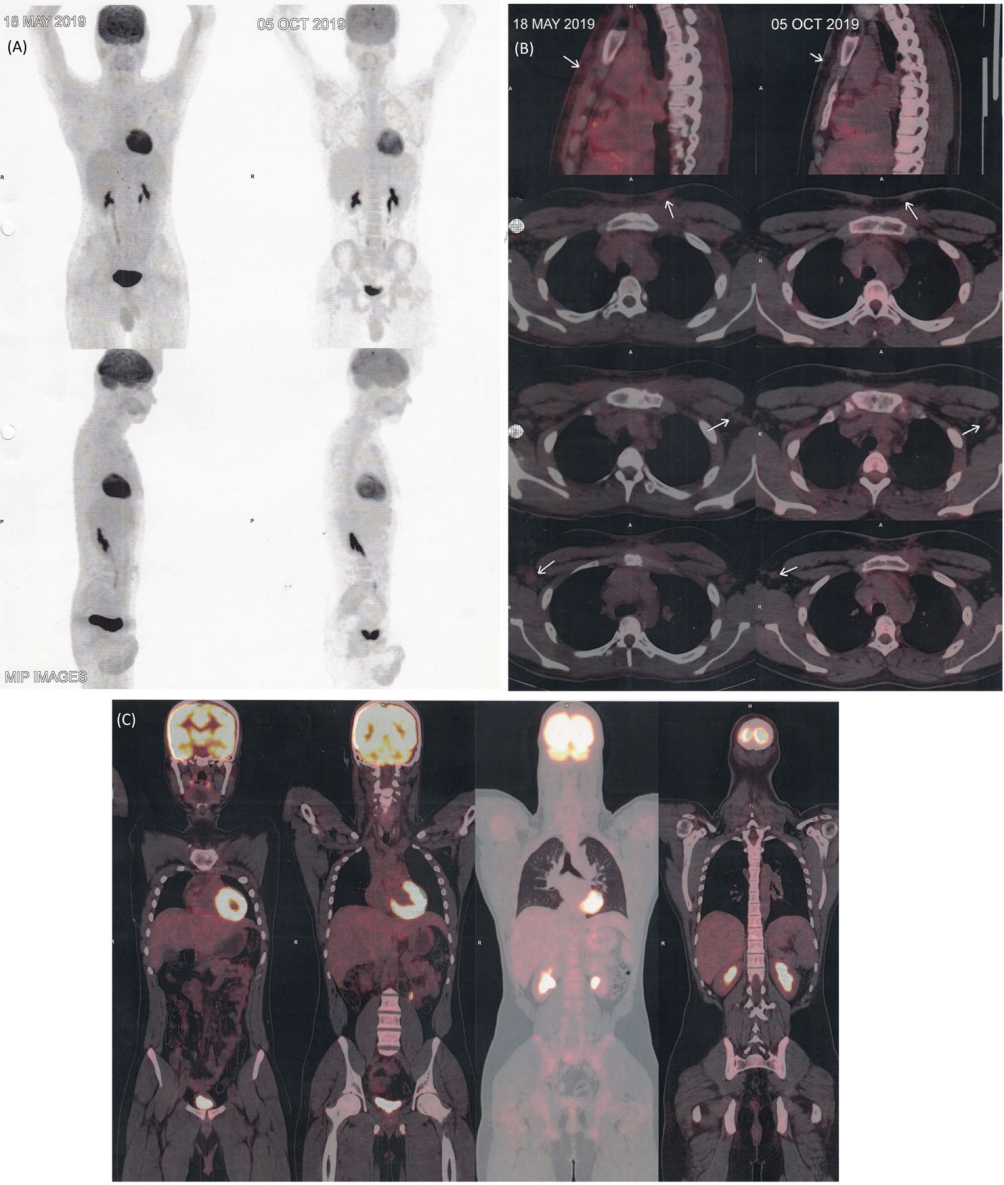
Comparative positron emission tomography‐computed tomography (PET‐CT) and CT imaging during 3 cycle of ABVD and 1 month after 6 cycle of ABVD. (A) A whole body scan showing regression of the primary pathology involving the lymph node (left) and PET scan done after 6 cycle of ABVD showing regression in size with resolution of metabolic activity of supraclavicular, axillary and mediastinal nodes (right). (B–C) There is further regression in size of the supraclavicular, bilateral axillary, subpectoral, deep pectoral, bilateral internal mammary, and perivascular nodes are seen
